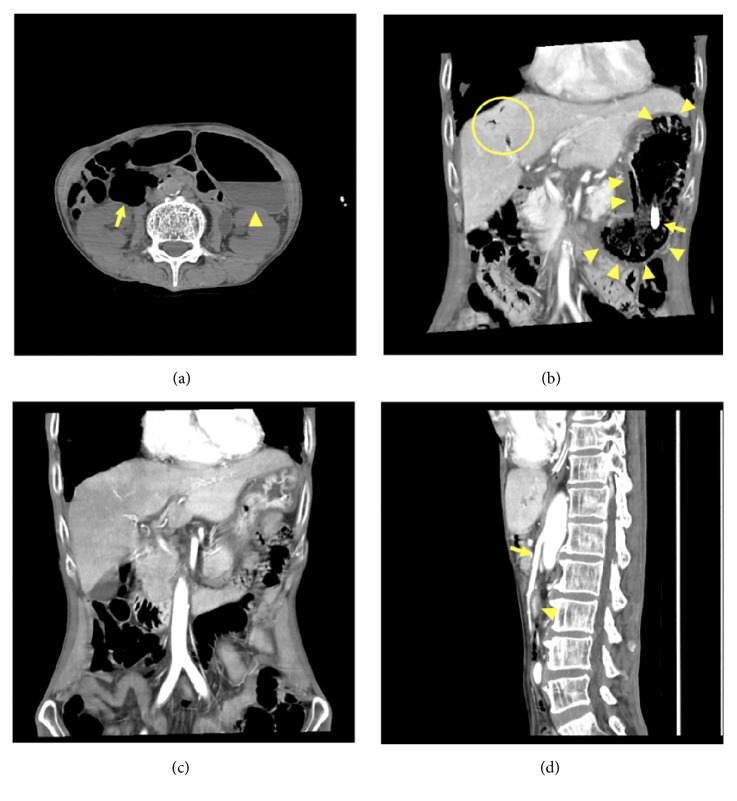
Plain abdominal CT scan on admission showing dilatation of the stomach (arrowhead) and second portion of the duodenum (arrow) (a). Contrast-enhanced CT scan on hospital day 11 showing gas within the wall of a distended stomach (arrowheads) and portal vein (circled region). An arrow demonstrating the tip of the feeding tube (b). Follow-up CT scan showing improvement of gas in the stomach and portal vein (c). Sagittal view showing an acute angle of the aorto-superior mesenteric artery (arrow) and a collapsed duodenum (arrowhead) (d).
